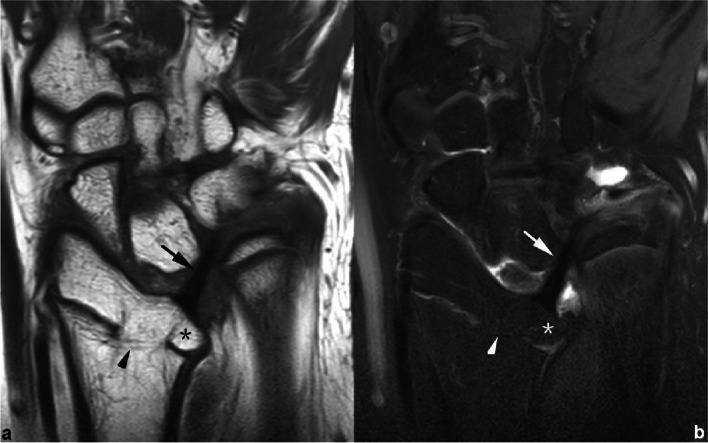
Left wrist MRI of the patient No. 0512. a Coronal T1WI image and b) coronal PDWI-FS image show the radial attachment of CD (black/white arrow) was located at the distal articular surface level (black/white *) without proximal displacement. No definite VL and/or RTL were observed in this case. Hence, patient No. 0512 should be diagnosed with Madelung-like deformity, caused by the local premature closure region (black/white arrowhead) of the distal radial growth plate
